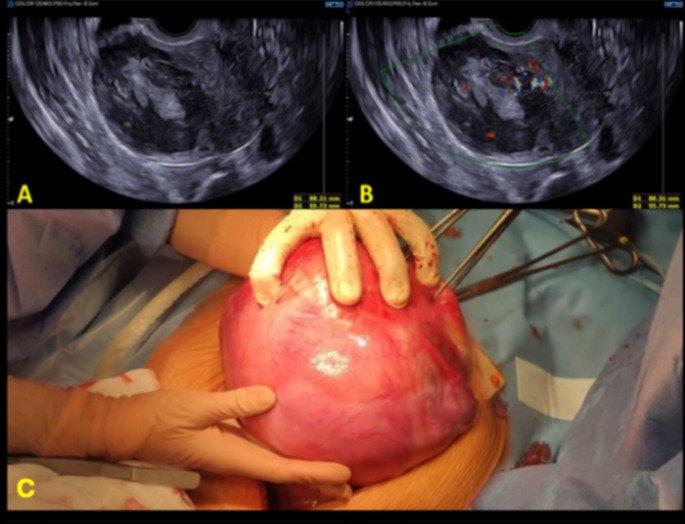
Transvaginal ultrasound shows a single large lesion (≈ 9 cm) with well-defined margins and a heterogeneous echotexture due to the presence of hypoechoic areas, likely of cystic-degenerative nature (A), with visible vascularization on Doppler (B). During surgery, a fleshy mass was enucleated (C)
